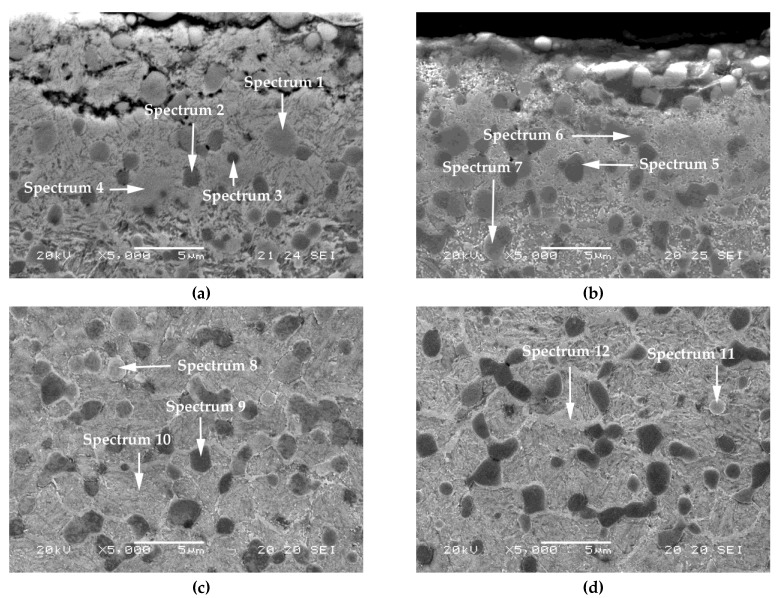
Phases of the nitrided layer, analyzed by energy dispersive X-ray (EDX) microanalysis; (a) Experiment 5; (b) Experiment 6; (c) Experiment 7; (d) Experiment 8.
